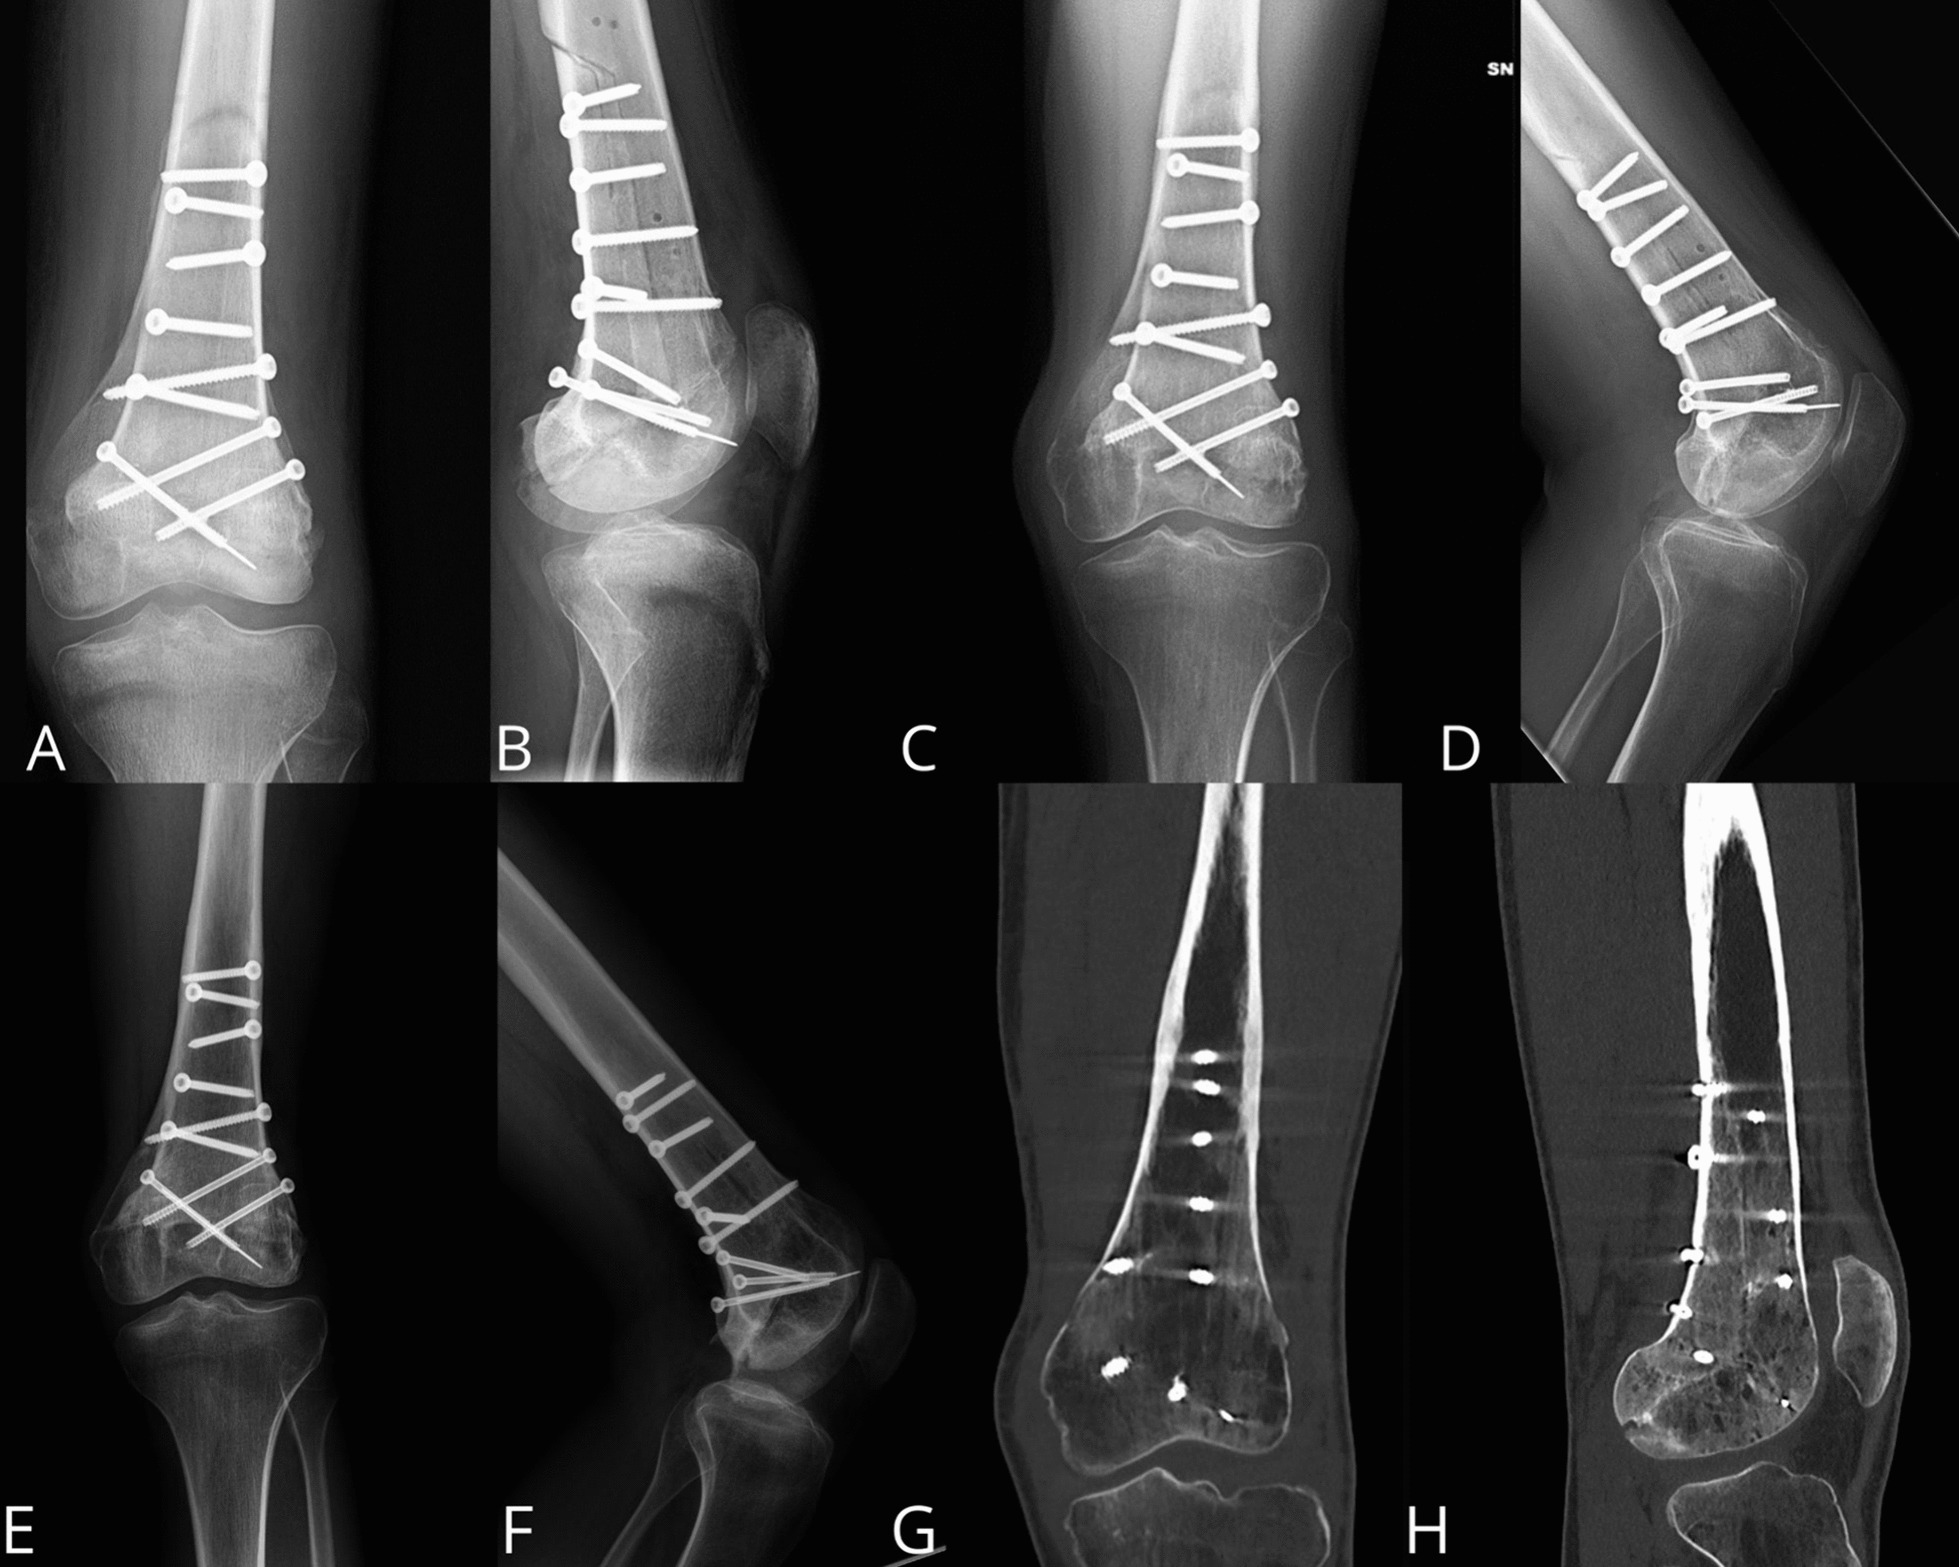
A, B: Antero-Posterior and Lateral X-rays at 3 months; C, D: Antero-Posterior and Lateral X-rays after 9 months: the graft is almost completely fused to the host bone; E, F: Antero-Posterior and Lateral X-rays at 12 months: the graft is perfectly integrated; G, H: CT scan at 18 months, the graft is perfectly integrated
